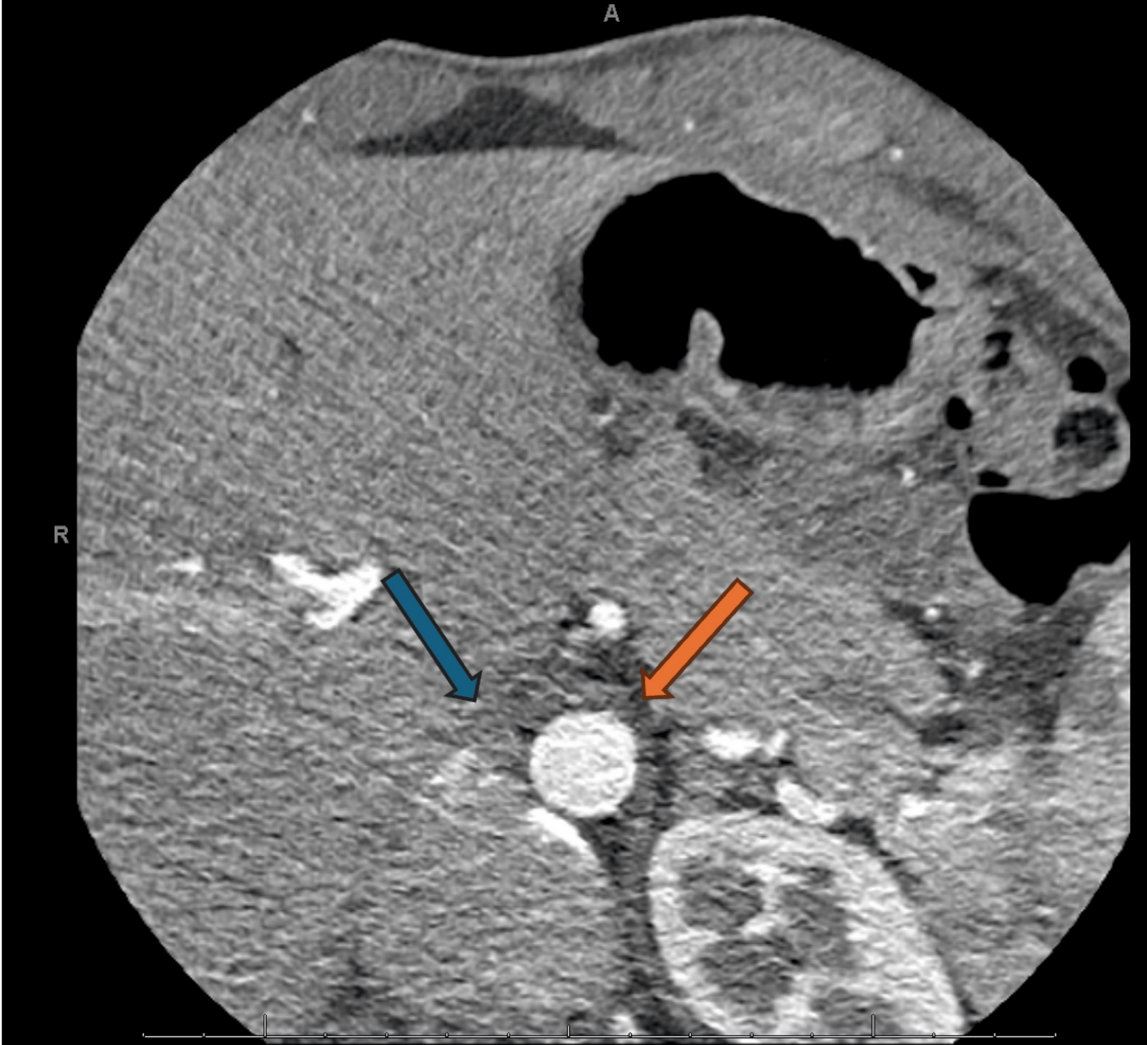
CT coronary angiogram: axial view at the abdomen level (liver), showing the aorta, and discontinuation of the inferior vena cava The blue arrow shows the discontinuation of the inferior vena cava at the abdominal level. The orange arrow shows the descending aorta for reference. These imaging findings were critical in guiding the decision to attempt a retrograde aortic approach for ablation, as the abnormal venous anatomy complicated standard access.
